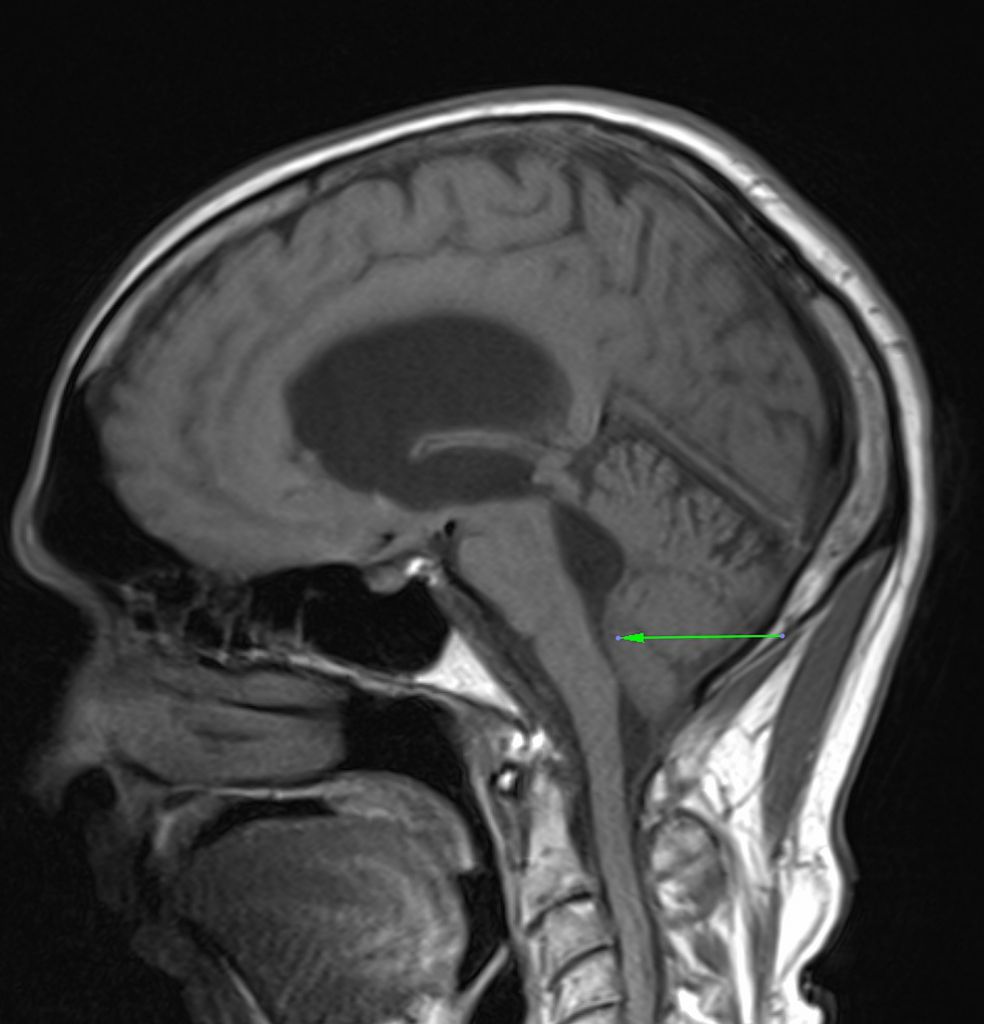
Sagittal T1-weighted MRI showing dilatation of the ventricular system, with stenosis at the floor of the fourth ventricle (arrow)
